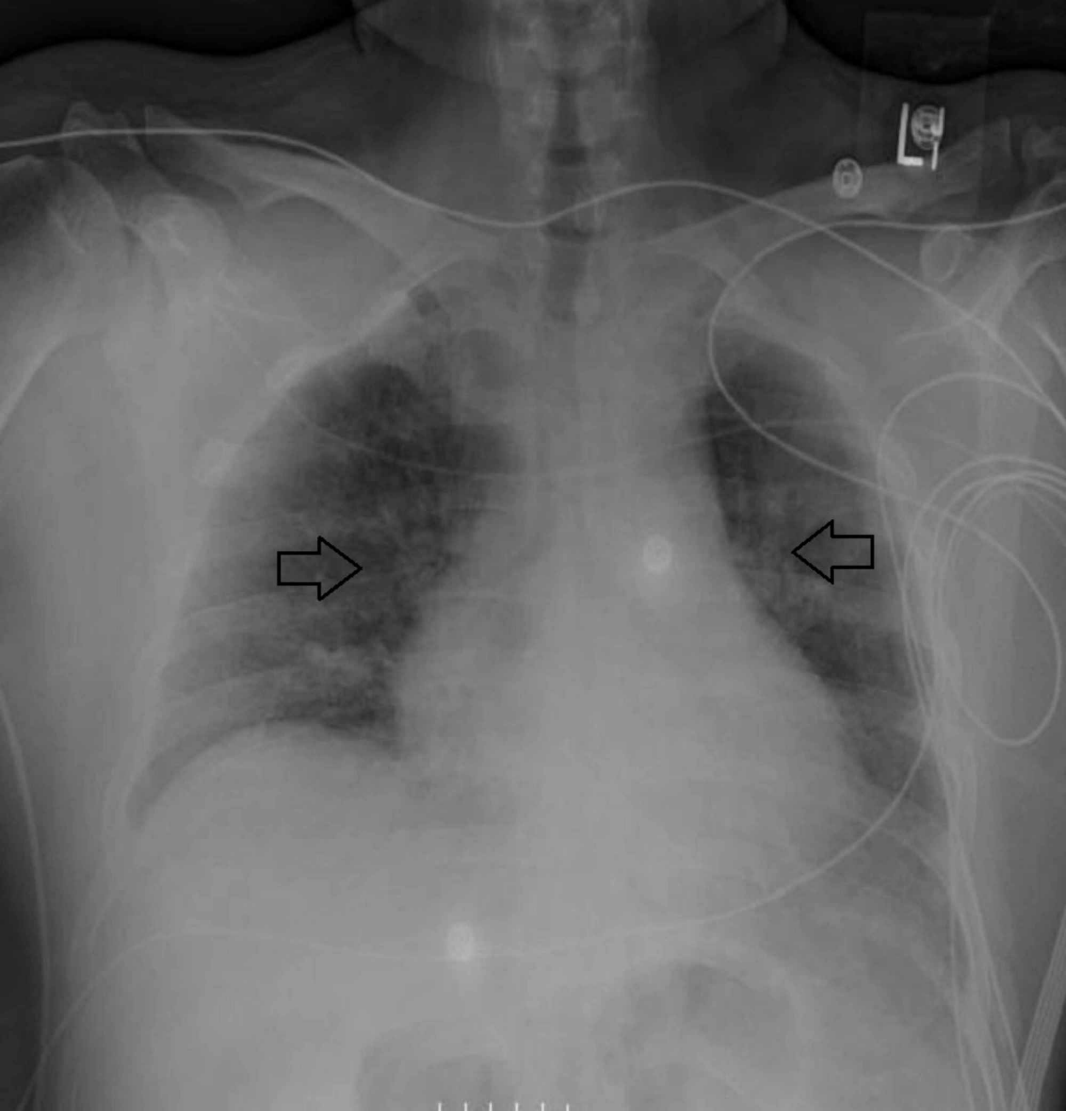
Anteroposterior (AP) chest X-ray showing interval decrease in bilateral lung infiltrates seven days after initiation of extra-corporeal membrane oxygenation (ECMO) and addition of pulse-dose steroids.
